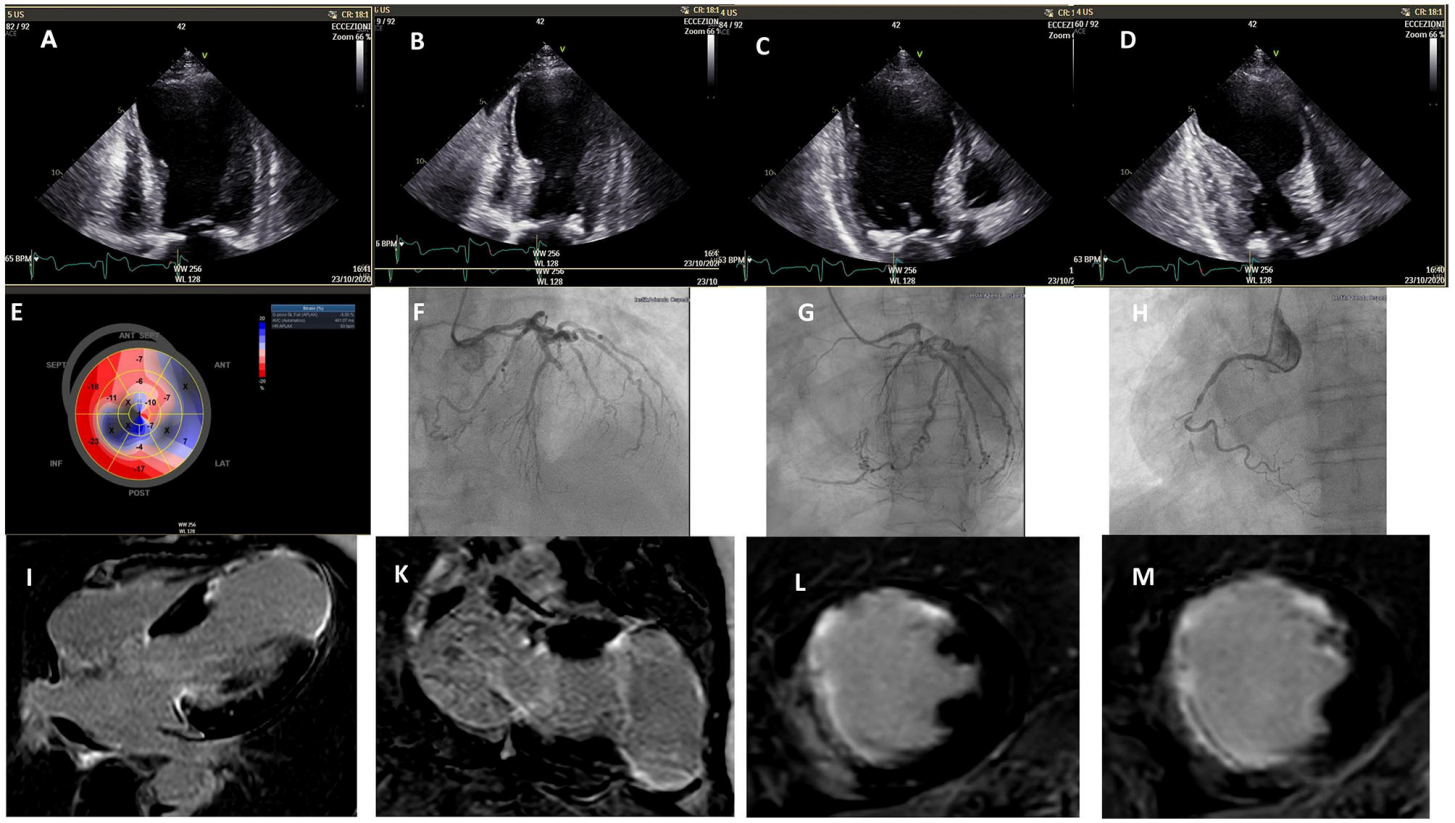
2D echo: (A–D) left ventricular aneurysm: systolic and diastolic phase; (E) baseline global longitudinal strain; coronary angiograms; (F) occlusion of the proximal left anterior descending coronary artery; (G) circumflex artery; (H) occlusion of proximal right coronary artery; cardiac MRI; (I–M) transmural delayed enhancement in the myocardium wall supplied by LAD.
